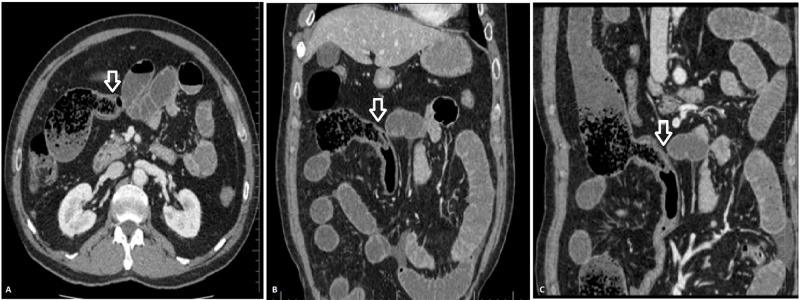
MDCT axial (A), coronal (B), and sagittal (C) images showing inverted Meckel’s diverticulum (arrow) resulting in small bowel obstruction with small bowel feces sign in the distal ileum MDCT: multidetector computed tomography
